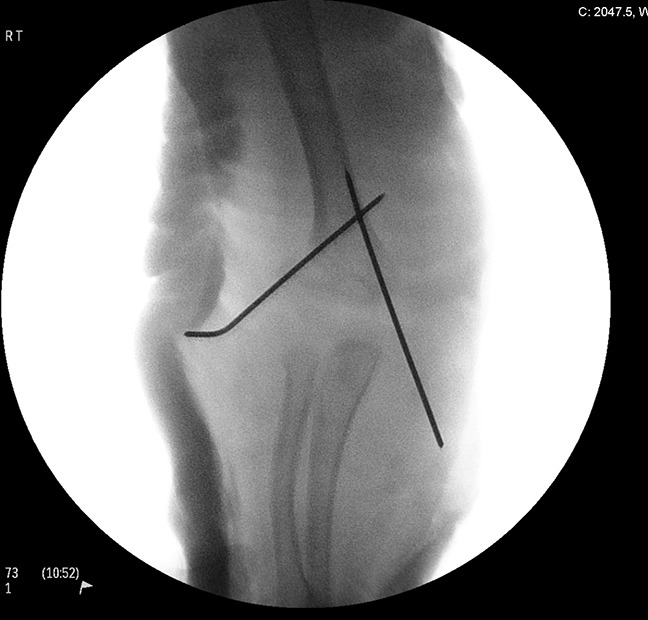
Radiograph showing immediate postsurgery position
